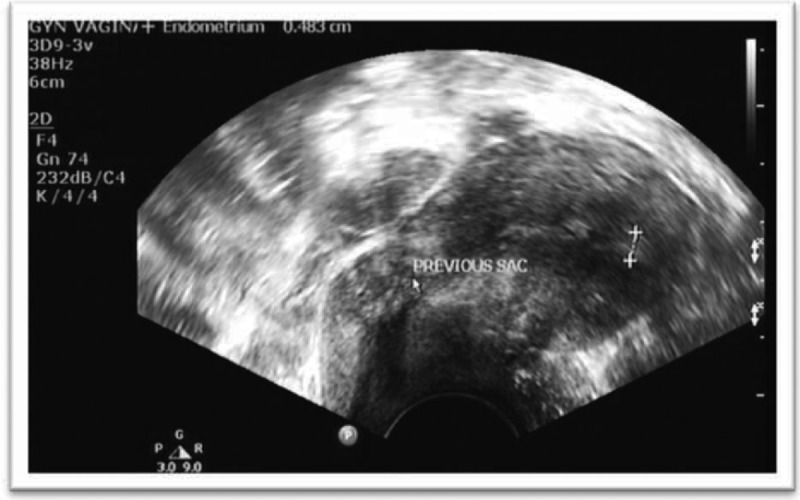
Transvaginal ultrasound (TVUS) demonstrating a normal uterus with no embryonic signs in the place of the previous ectopic sac.
